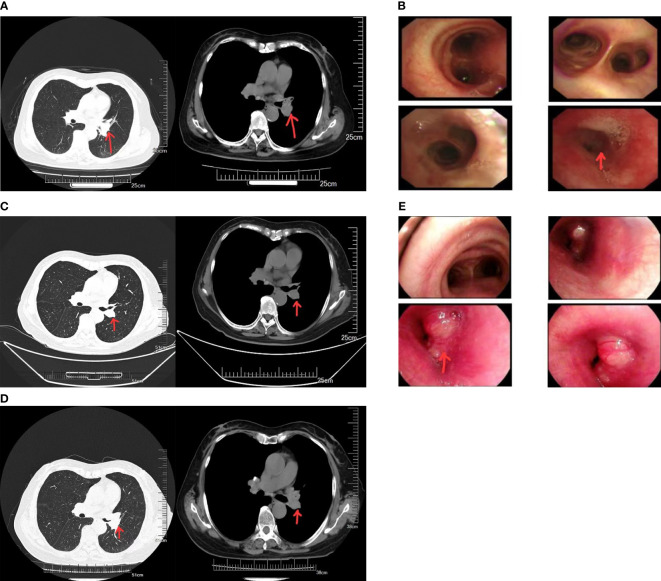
(A) Computed tomography (CT) images of the patient before neoadjuvant therapy. (B) Results of the first bronchoscopy. (C) CT images of the patient after three cycles of sintilimab immunotherapy. (D) CT images of the patient after five cycles of sintilimab immunotherapy. (E) Results of the second bronchoscopy.
